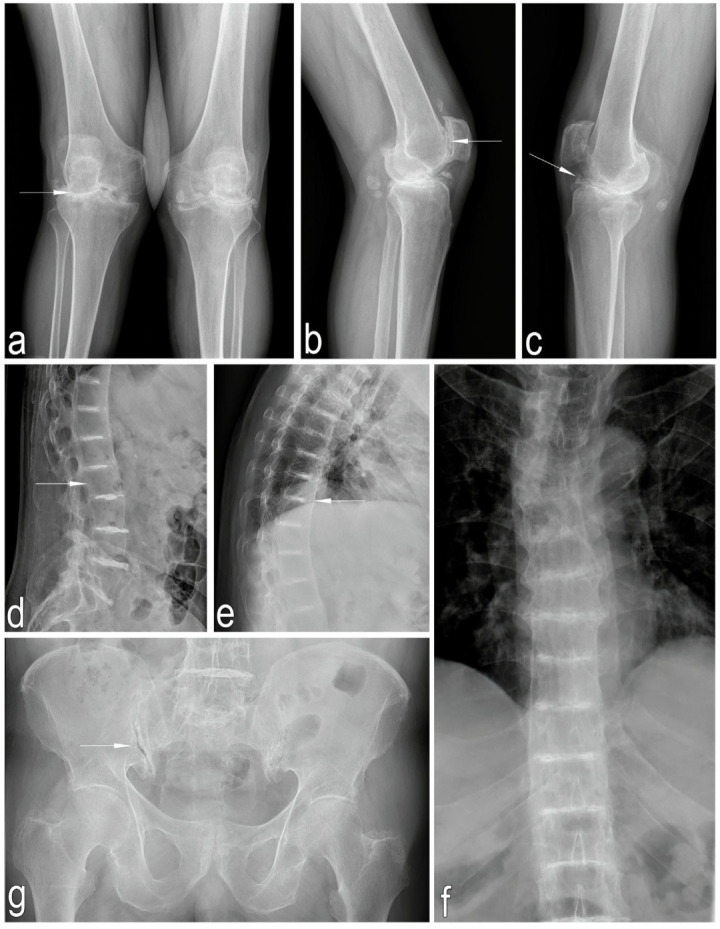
(a)—Anteroposterior (AP) X-rays of the knees in standing position, arrows—constriction of the articular fissure and osteophytic changes; (b,c)—Lateral views of the knees, arrows—joint free bodies and OA changes in patellofemoral joints; (d–f)—Thoracic and lumbar spine X-rays in AP and lateral views, arrows—osteoporotic bones and multilevel intervertebral disc calcification; (g)—AP views of the pelvis and hip joints, arrows—osteophytic changes in sacroiliac joint.
